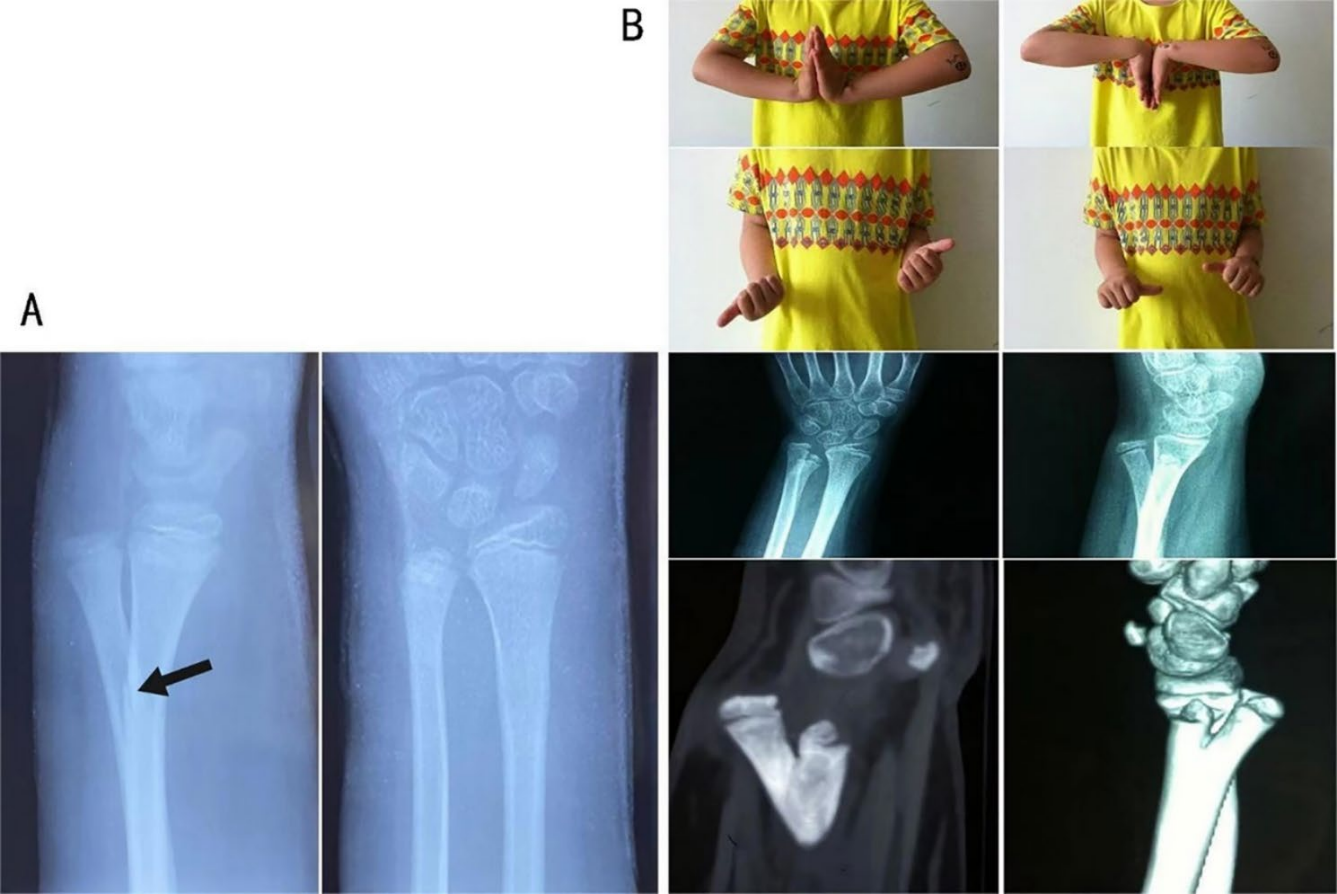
(A) X-ray of the patient at the time of injury, greenstick fracture of the left radius (Arrow) with possible dislocation of the distal ulna at the DRUJ. (B) The patient’s hand movement, X-ray and CT 3D reconstruction 2 months after the injury
